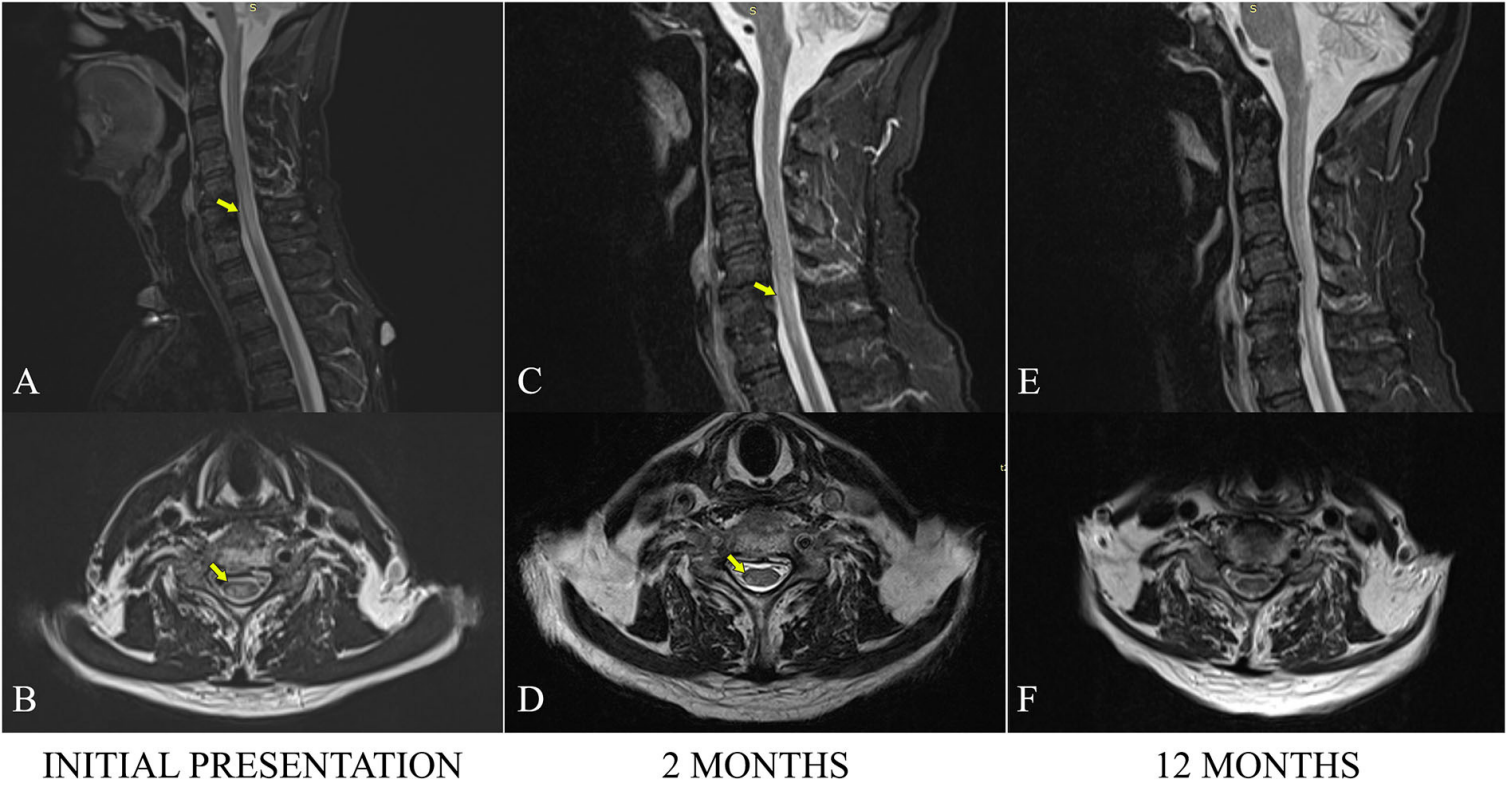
Sagittal MRI STIR sections of the cervical spine at admission (A), 2 months (C), and 12 months (E). Axial MRI TSE sections at approximatively the same level (a plane passing through the upper C6 plateau) at admission (B), 2 months (D), and 12 months (F). The hyperintense STIR cervical lesion is visible, extending from C4 to C7. Progressive fading of the increased signal is visible over time, especially on the axial images.
